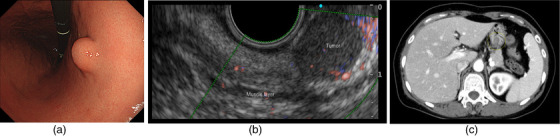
(a) The submucosal tumor (12×12 mm) located in the lesser curvature side of the stomach. (b) Endoscopic ultrasound showed an irregular low‐echoic lesion connected to the muscle layer. (c) Computed tomography showed a low‐density mass in the stomach. The tumor size was 12 × 12 mm. There was no tumor metastasis.
